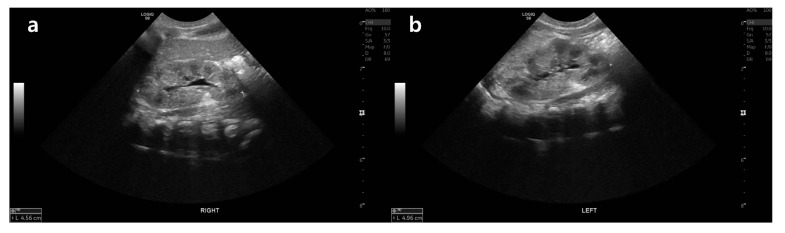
Renal ultrasonography of the patient. (a) Mild hydronephrosis with grade 1 pelvic dilatation in the right kidney. (b) No abnormal findings in the left kidney.
